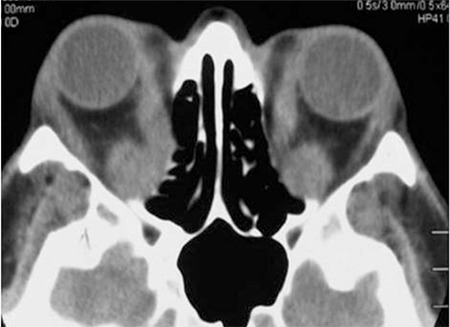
Orbital computed tomography images showing enlarged inferior and medial rectus muscles in a patient with thyroid-associated ophthalmopathy. The inferior rectus muscle is enlarged, mimicing an orbital tumor
